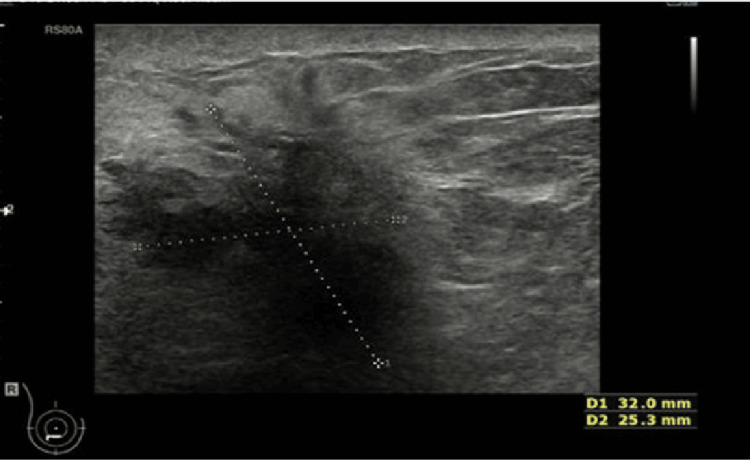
(Case 1) Right breast ultrasound scan reveals a highly suspicious 32 mm U5 lesion located in the lower outer quadrant of the right breast.
